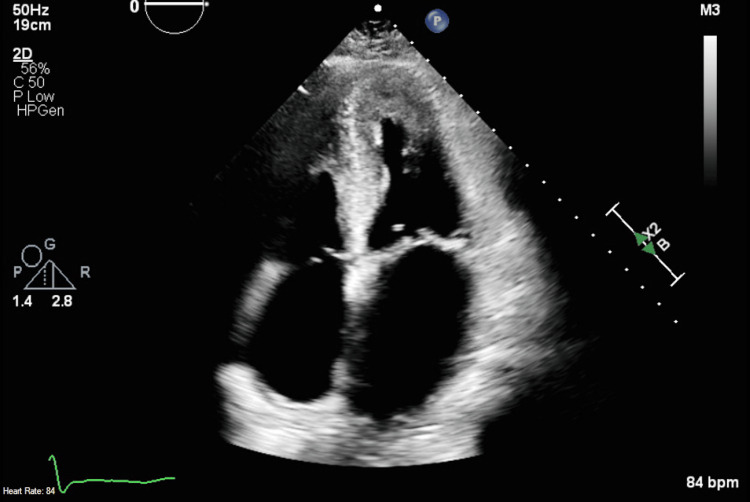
Apical four-chamber view echocardiogram showing left atrial dilation and left ventricular hypertrophy.
